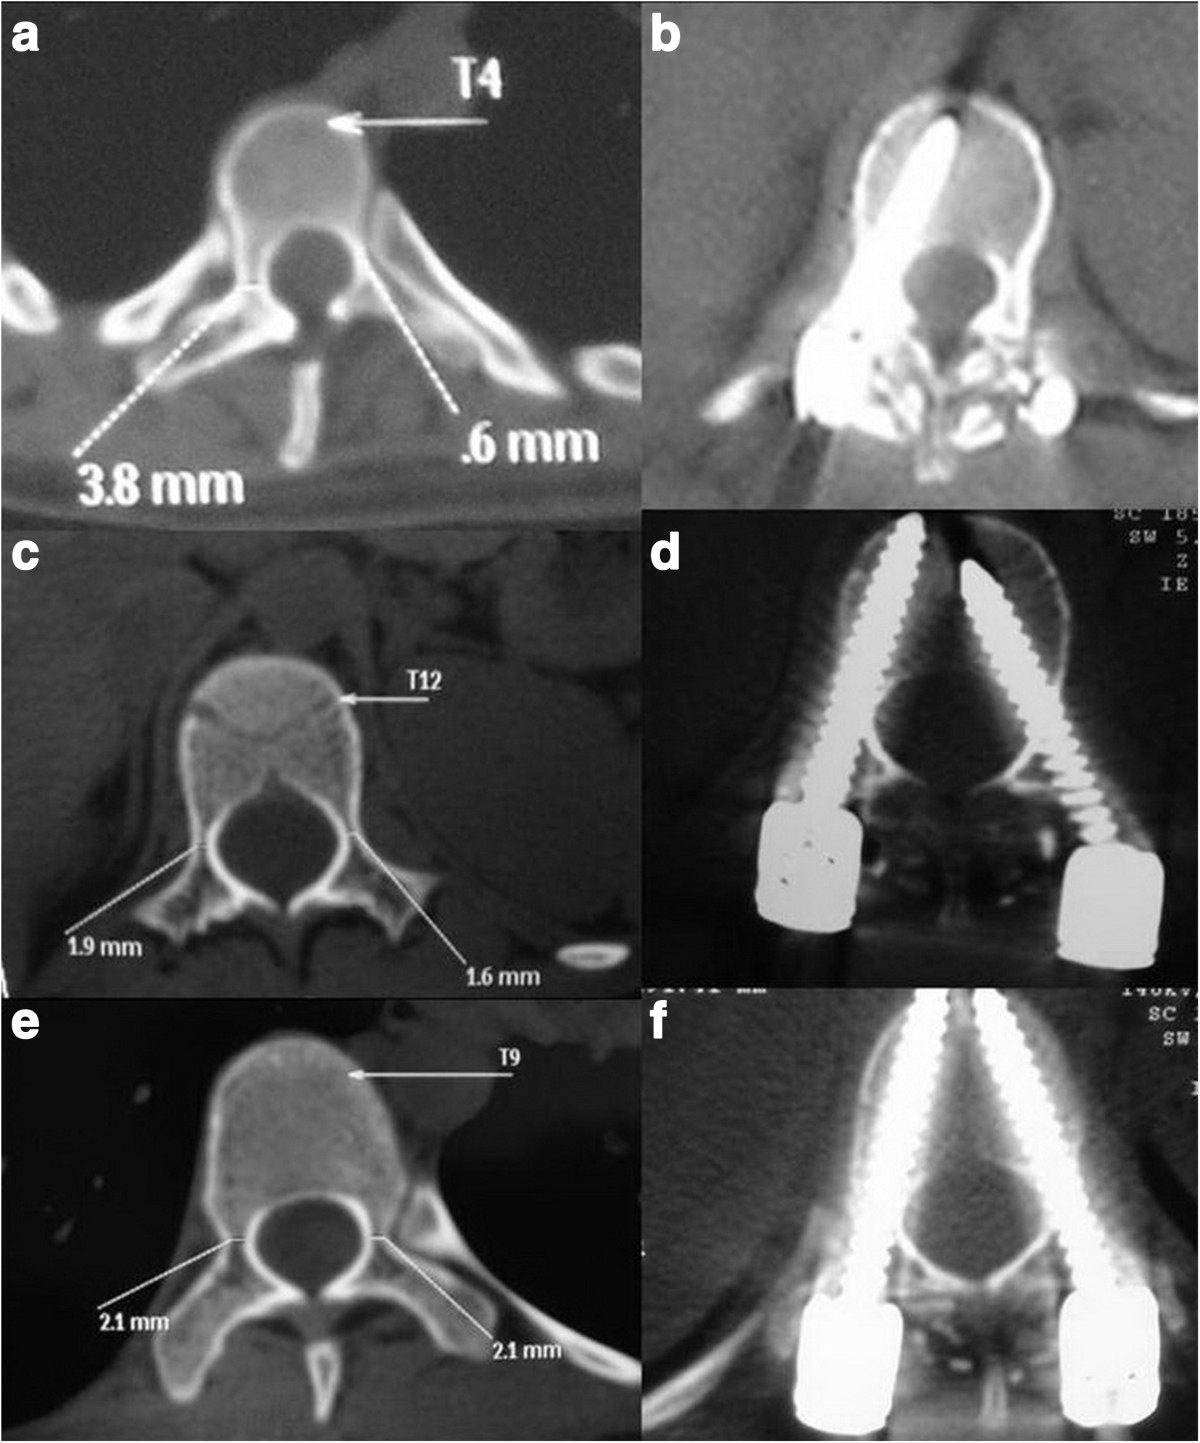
Preoperative and postoperative computed tomography images of pedicle classification determined by the inner cortical width. (a) Preoperative type I pedicle. (b) Postoperative type I pedicle. (c) Preoperative type IIa pedicle. (d) Postoperative type IIa pedicle. (e) Preoperative type IIb pedicle. (f) Postoperative type IIb pedicle.
